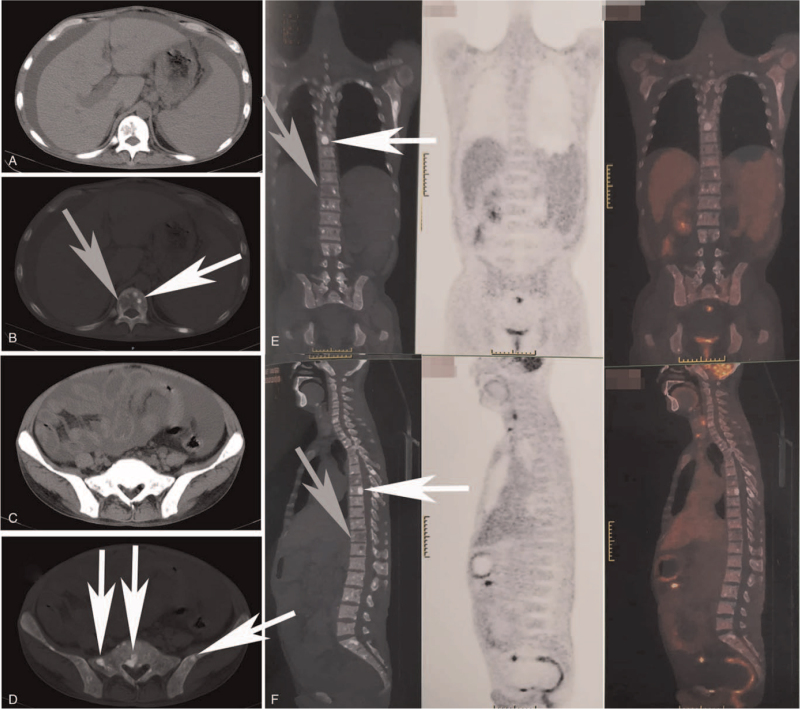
A–D. CT scan showed hepatosplenomegaly and ascites (A), mixed lesions with osteosclerosis and osteolysis in lumbar vertebrae (B), ascites and swelling bowels (C), and osteosclerosis in sacrum and ilium (D) (The white arrows direct the osteosclerosis and the grey arrows direct the osteolysis.). E and F. PET/CT showed mixed bone lesions in spinal column with normal SUV of ^18^FDG (The white arrows direct the osteosclerosis and the grey arrows direct the osteolysis.). CT = computed tomography, PET = positron emission tomography, SUV = standard uptake value.
